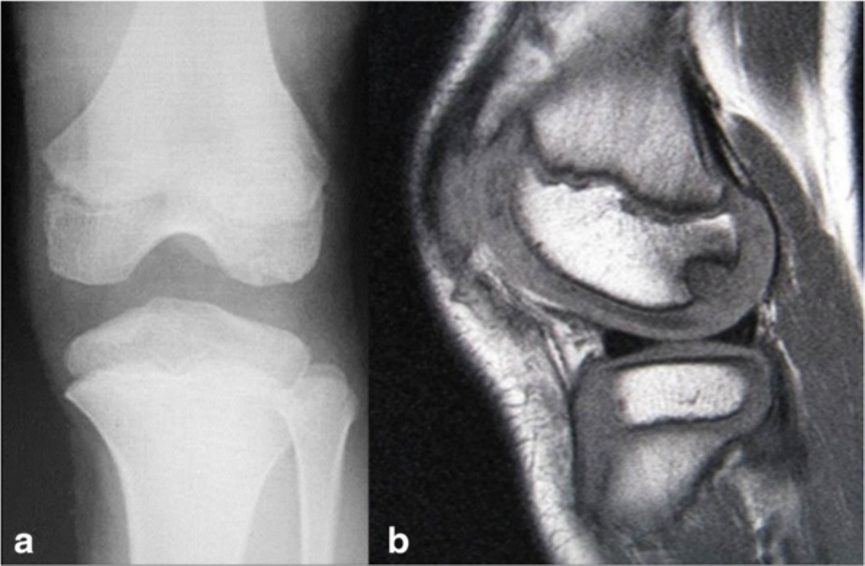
Image examinations at 6 months after the initial visit. a A posterior-anterior weight-bearing radiograph (Rosenberg’s view) of the patient’s left knee after 6 months of conservative treatment. b T1-weighted sagittal magnetic resonance imaging image. The osteochondritis dissecans lesion is evident
